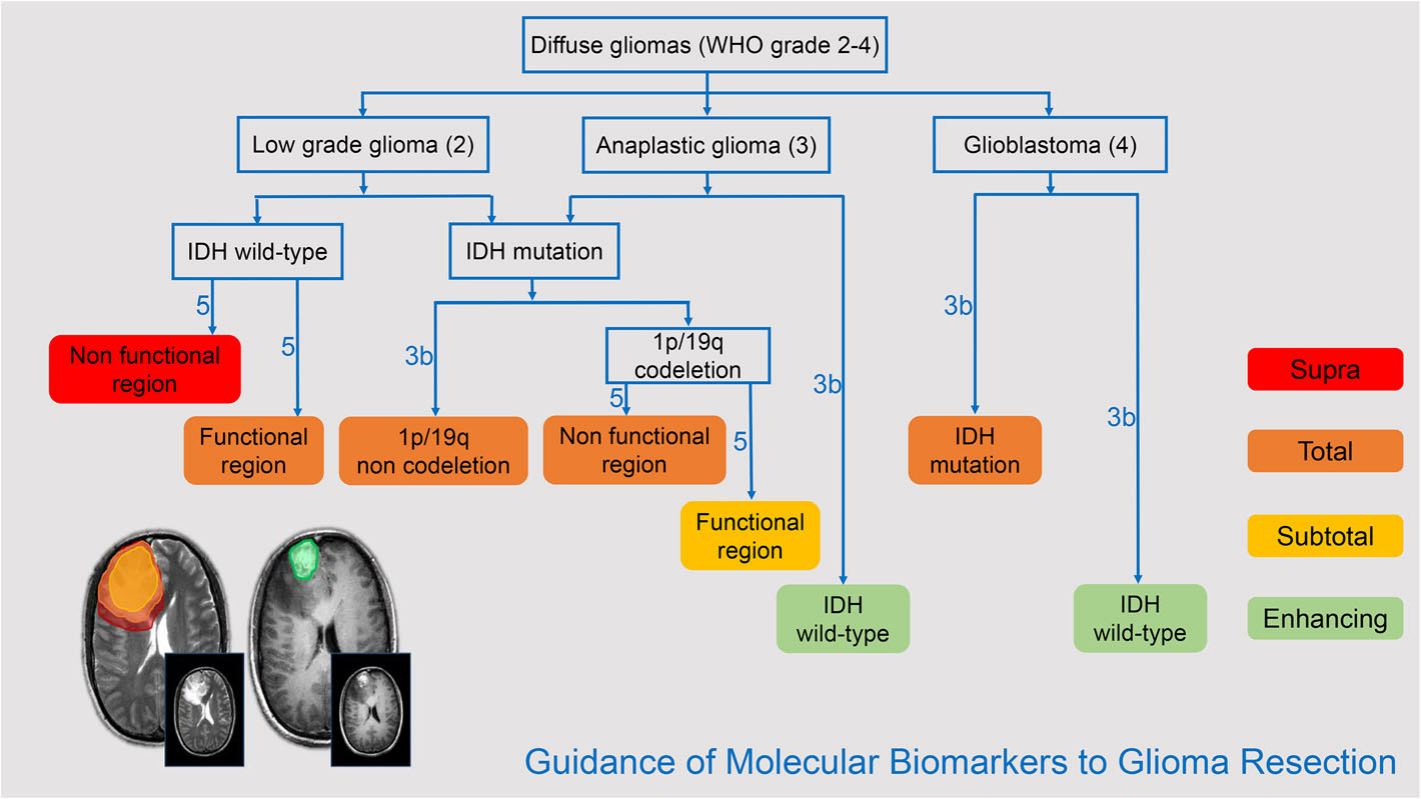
Guidance of molecular biomarkers to glioma resection. The figure shows the recommended extent of resection for diffuse gliomas according to pathological grade and molecular biomarkers. The axial T2-weighted image and contrast-enhanced T1-weighted image presented four levels of EOR as follows: red, supra-total resection; orange, total resection; yellow, subtotal resection; and green, enhancing tissue resection. Supra-total resection is recommended for low-grade diffuse gliomas with IDH wild-type in the non-functional region; subtotal resection is recommended for diffuse gliomas with IDH mutation and 1p/19q codeletion in the functional region; enhancing tissue resection is recommended for anaplastic gliomas and glioblastomas with IDH wild-type; total resection is recommended for the remaining subtypes of diffuse gliomas. The recommended level is determined according to the Oxford Centre for Evidence-based Medicine Levels of Evidence
